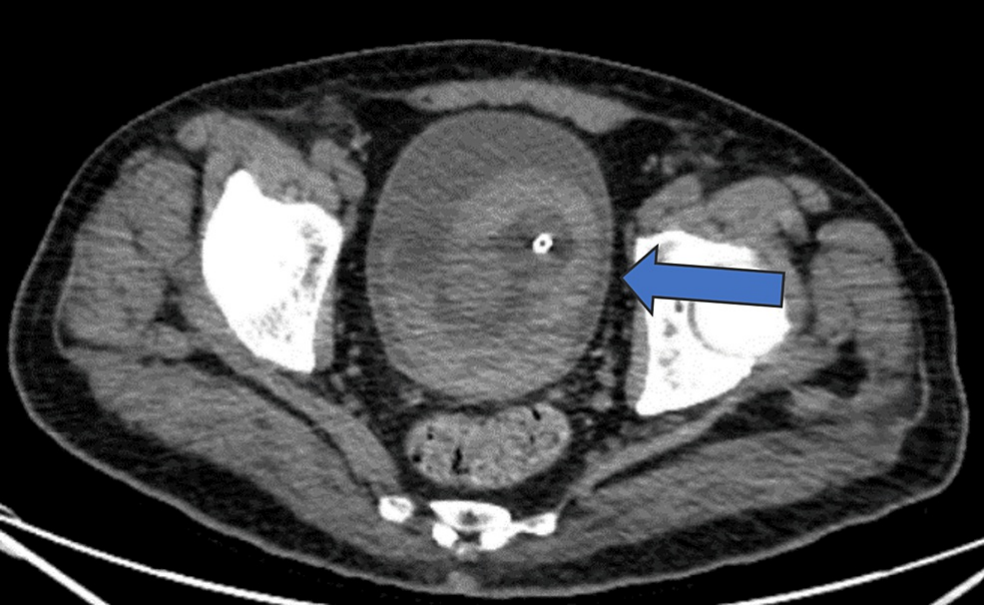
a CT scan of the abdomen and pelvis showing a sizable bladder hematoma
